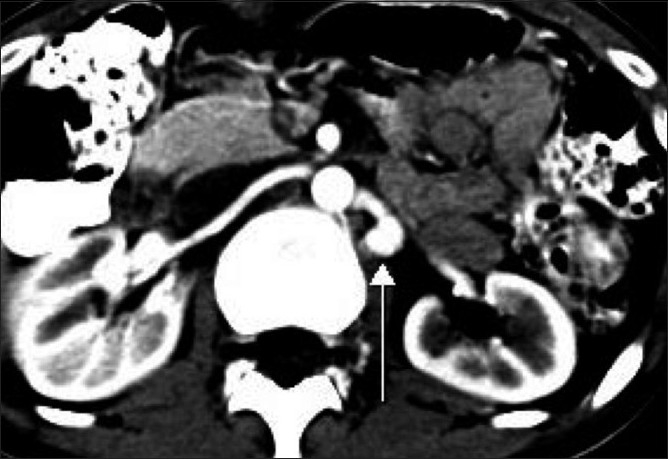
Axial contrast-enhanced CT scan shows homogeneous contrast enhancement within the structure that was overlapping the left renal artery
